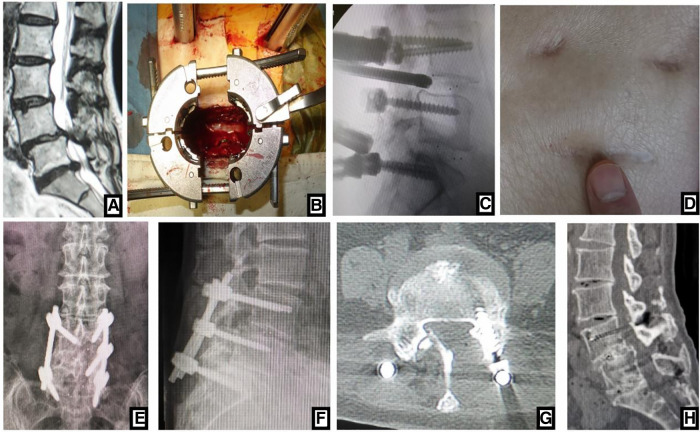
(A) sagittal MRI showed 2-level LDD (L3–L5) in a 72-year-old woman with neurologic symptoms. (B) Contralateral bridge Freehand MIPS combined with unilateral MIS-TLIF through tube was performed. After the cage was inserted into the intervertebral space through the tube, (C) the fluoroscopic image confirmed the position of cage. (D) The picture showed the incision like smile face, so this MIS-TLIF is called smile-face surgery. On (E, F) postoperative x-ray the position of pedicle screws and cages was good and (G) axial CT scan confirmed that the neurologic decompression was complete. On (H) sagittal CT at 5-year follow-up, fusion of grade I was achieved.
